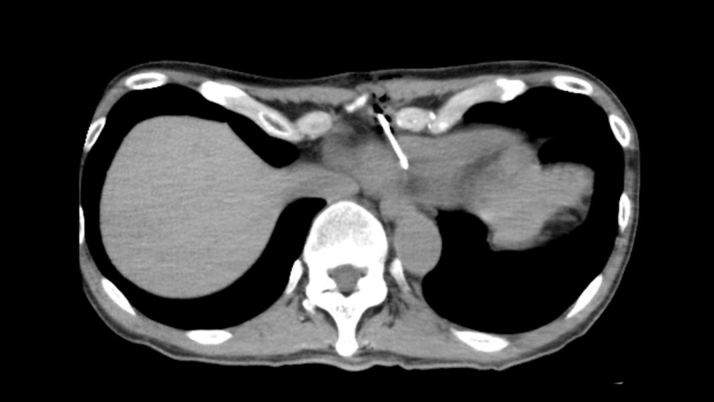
Computed Tomography–Guided Biopsy of Pericardial Tumor Under local anesthesia, 3 samples were obtained using an 18-gauge biopsy needle via the left lower parasternal approach. The procedure was completed without any complications.
